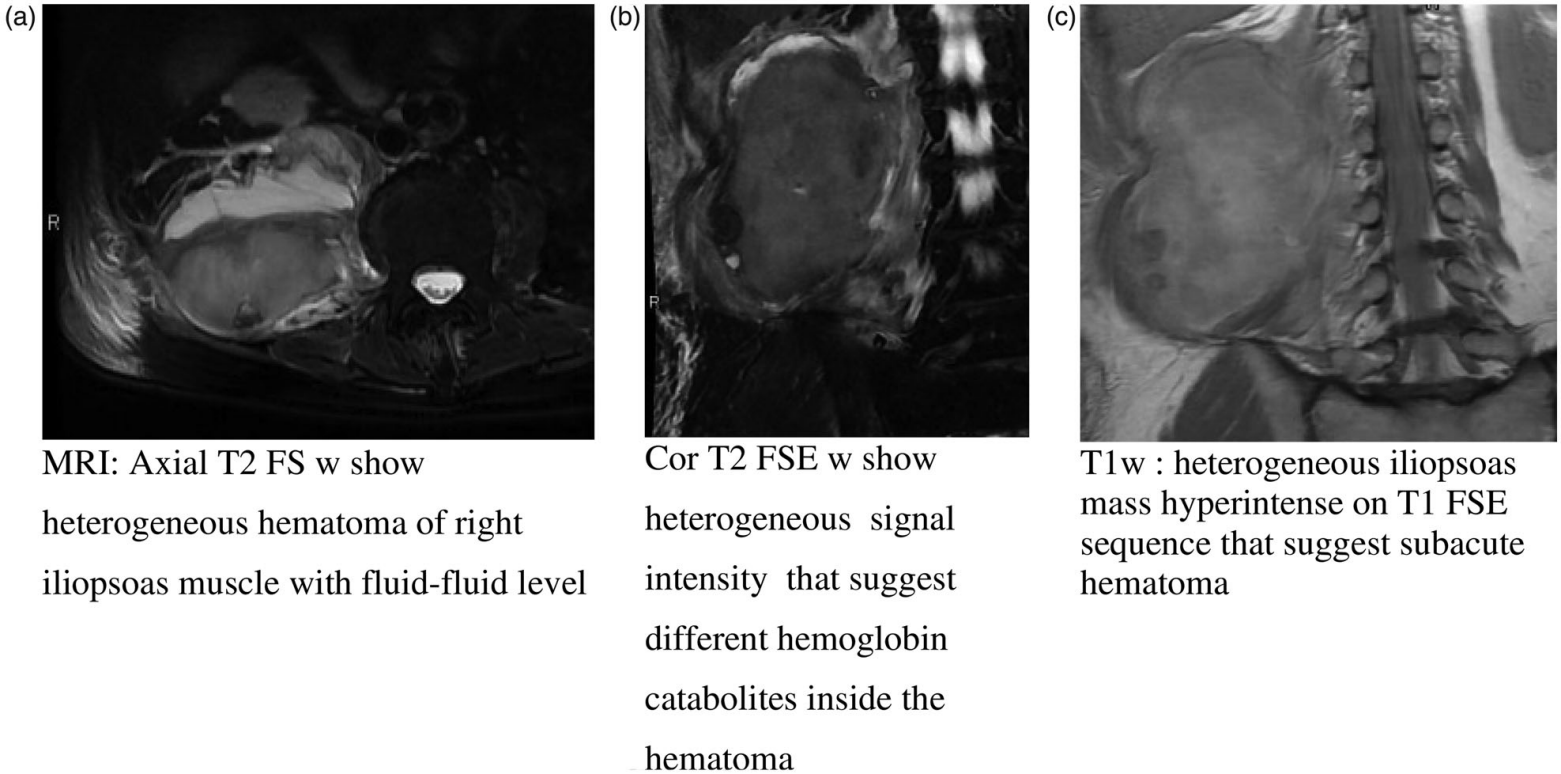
(a) MRI of right iliopsoas haematoma, (b) MRI of right iliopsoas haematoma and (c) MRI of right iliopsoas haematoma.
